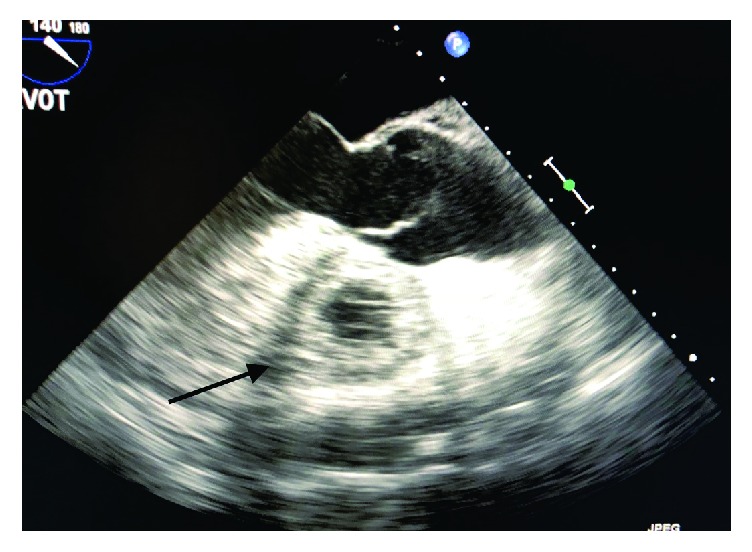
Transesophageal echocardiogram with ME AV LAX view showing extensive thrombus formation in the right ventricle.
